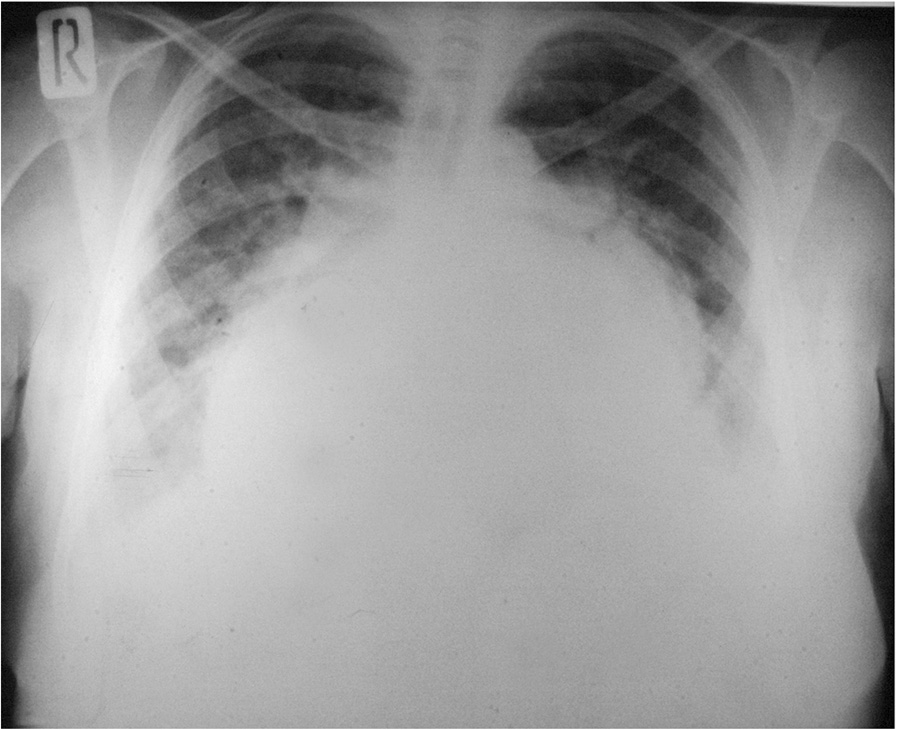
Preoperative chest x-ray showing marked cardiomegally and widening of the carina.
